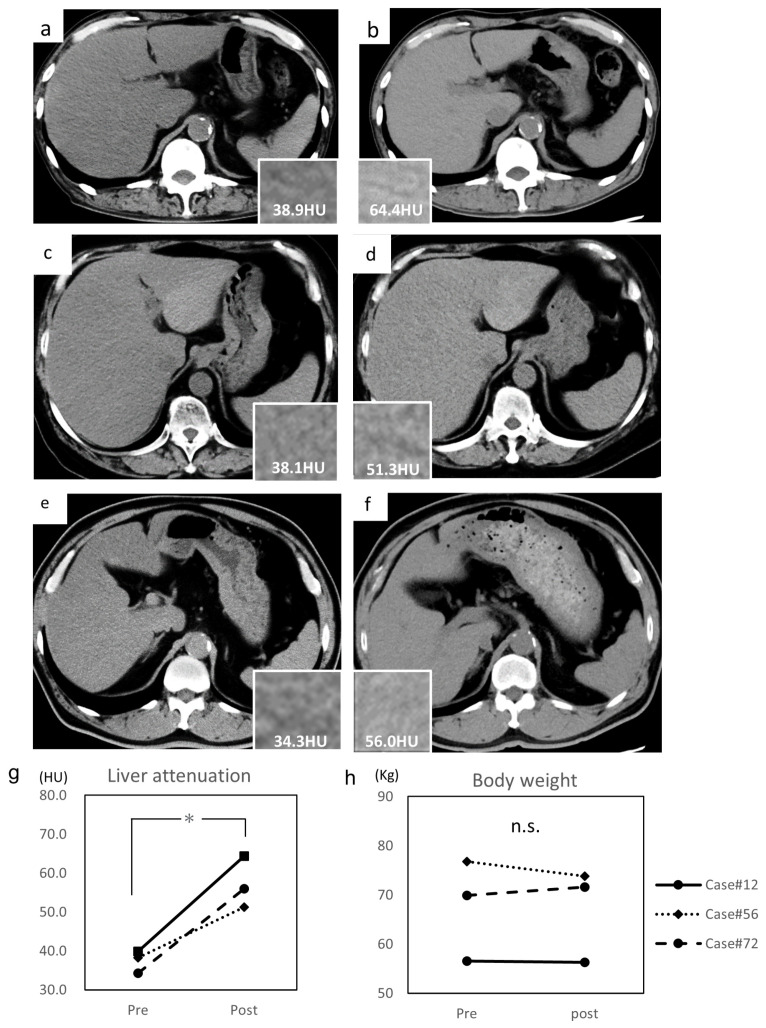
Alteration of liver attenuation assessed by CT imaging. (a) Pre-treatment abdominal CT of Case #12, (b) post-treatment abdominal CT of Case #12, (c) pre-treatment abdominal CT of Case #56, (d) post-treatment abdominal CT of Case #56, (e) pre-treatment abdominal CT of Case #72, and (f) post-treatment abdominal CT of Case #72. (g) Alteration of liver attenuation assessed by CT imaging before and after treatment. Liver attenuations are indicated in each column. (h) Alteration of body weight before and after treatment. Hounsfield unit, HU. Data are expressed as mean with standard error of the mean (SEM). n.s. not significant, * *p* < 0.05.
